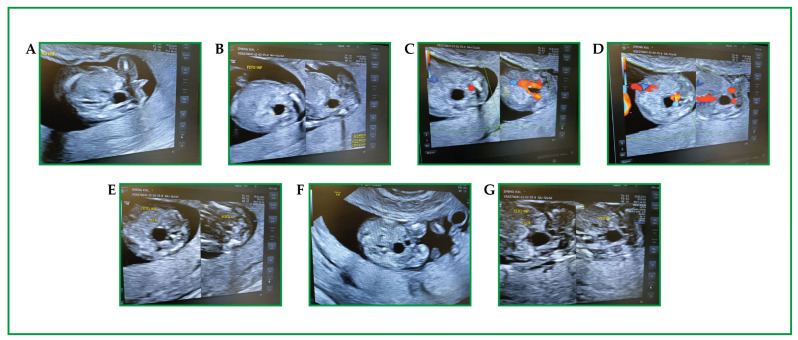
(A–G) First-trimester obstetric ultrasound in a twin pregnancy (GA 12+3): 2D and Color Doppler images focused on the fetal lower abdomen/pelvis. In both fetuses, an anechoic cystic structure is identified in the abdominopelvic region (labels “CISTI”), with smooth margins and no internal septations on the available frames. The fetal urinary bladder is visualized (labels “VESCICA”); however, based on static frames alone, the relationship between the cyst(s) and the bladder/urachus cannot be definitively established. On Color Doppler, no definite intralesional vascularity is demonstrated, while color signals are attributable to adjacent vascular structures. In one fetus, the lesion measures approximately 0.49 × 0.40 × 0.41 cm (three-axis calipers).
